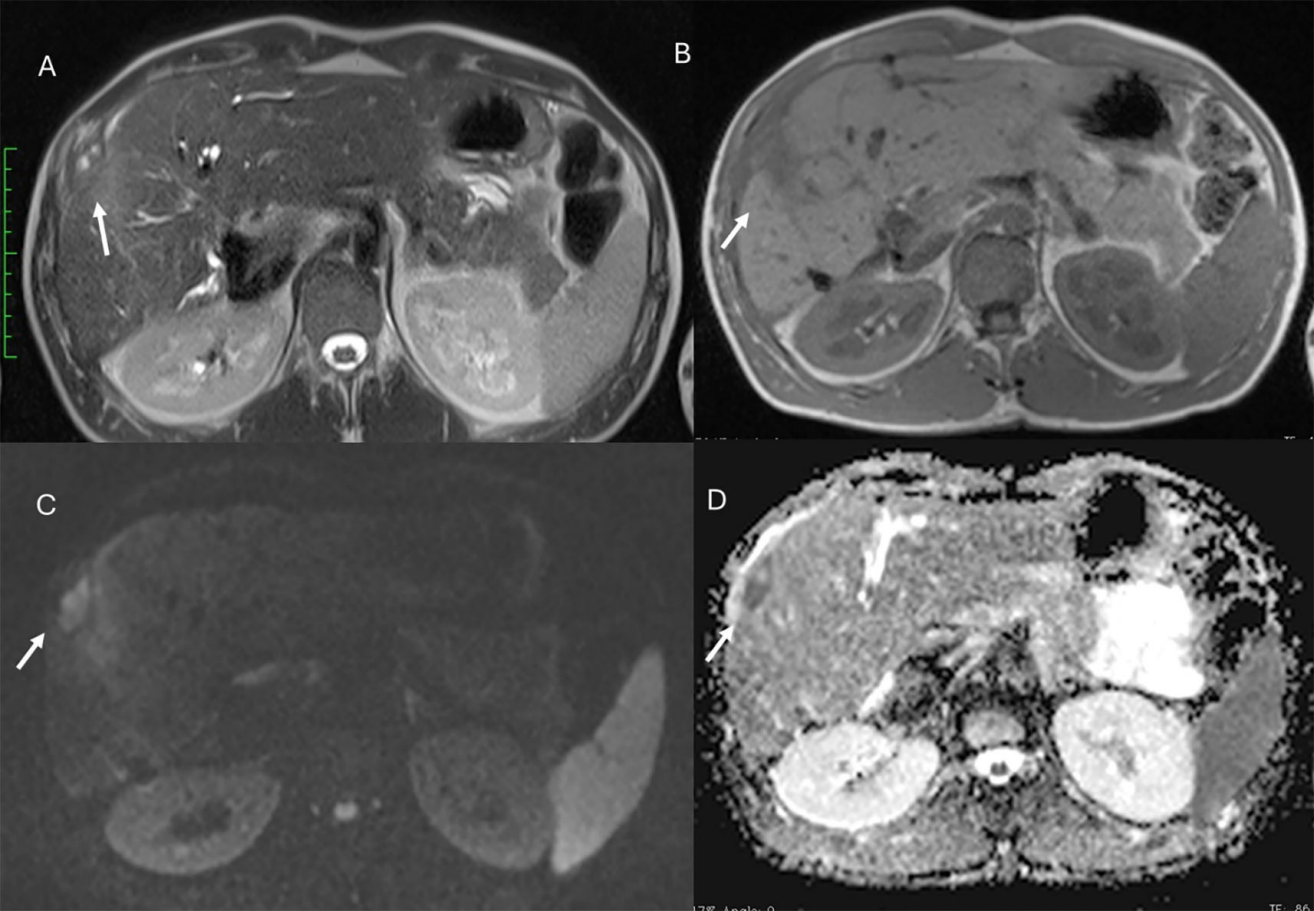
MRI of liver abscess after surgical resection. The lesion (arrows) shows hyperintense signal in T2-W sequence (A), hypointense signal in T1-W sequence (B), restricted diffusion on b = 800 s/mm² (C), and hypointense signal on ADC map (D). This demonstrates that a benign lesion can also show restricted signal and hypointensity on ADC maps.
